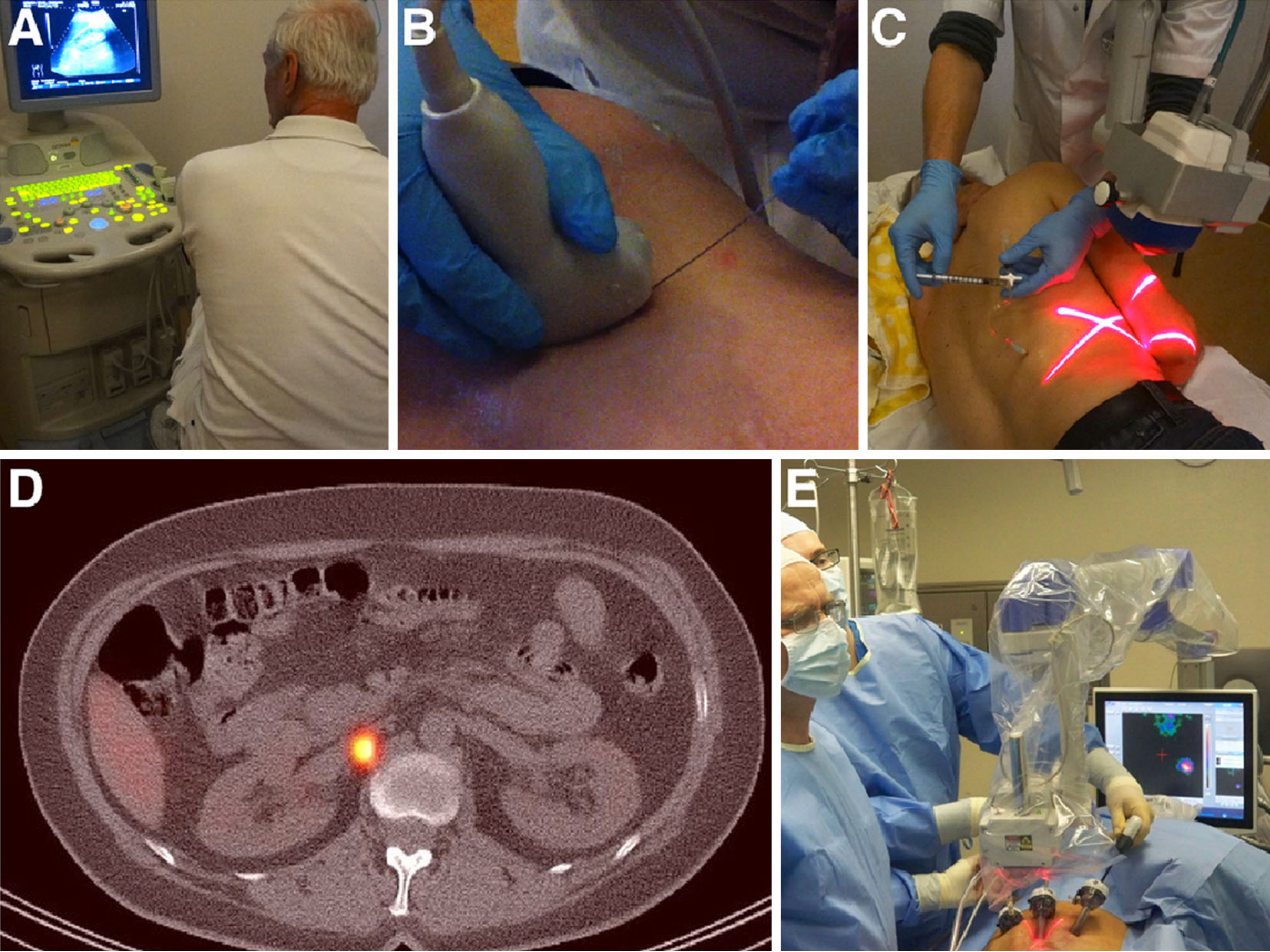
Sentinel node in renal-cell carcinoma. Following needle insertion under ultrasound guidance (a, b) ^99m^Tc-nanocolloid is administered into the tumour (c). Transversal SPECT/CT (d) shows a paracaval sentinel node, which is removed by means of laparoscopy assisted with a portable gamma camera (e)
